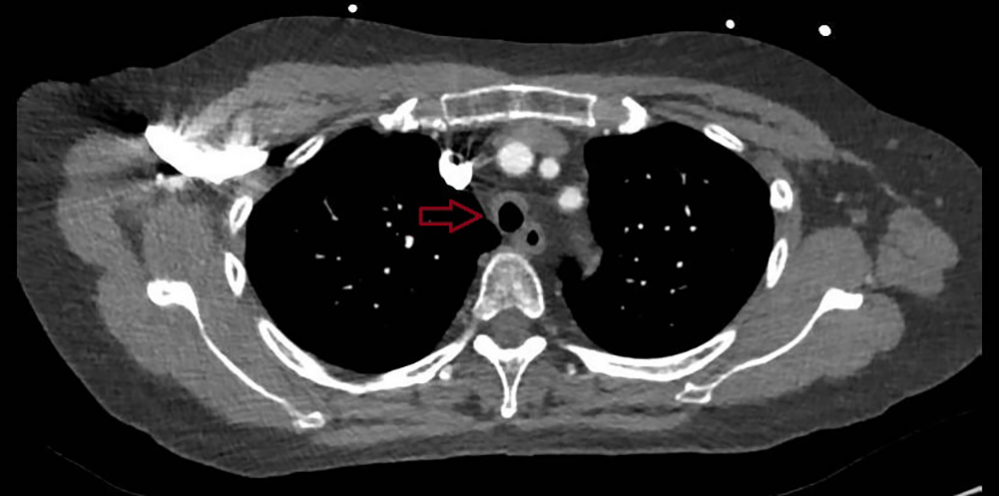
Computed tomography (CT) chest showing smooth thickening of cartilaginous portions of the trachea (anterior and lateral walls) with sparing of the posterior membranes.
